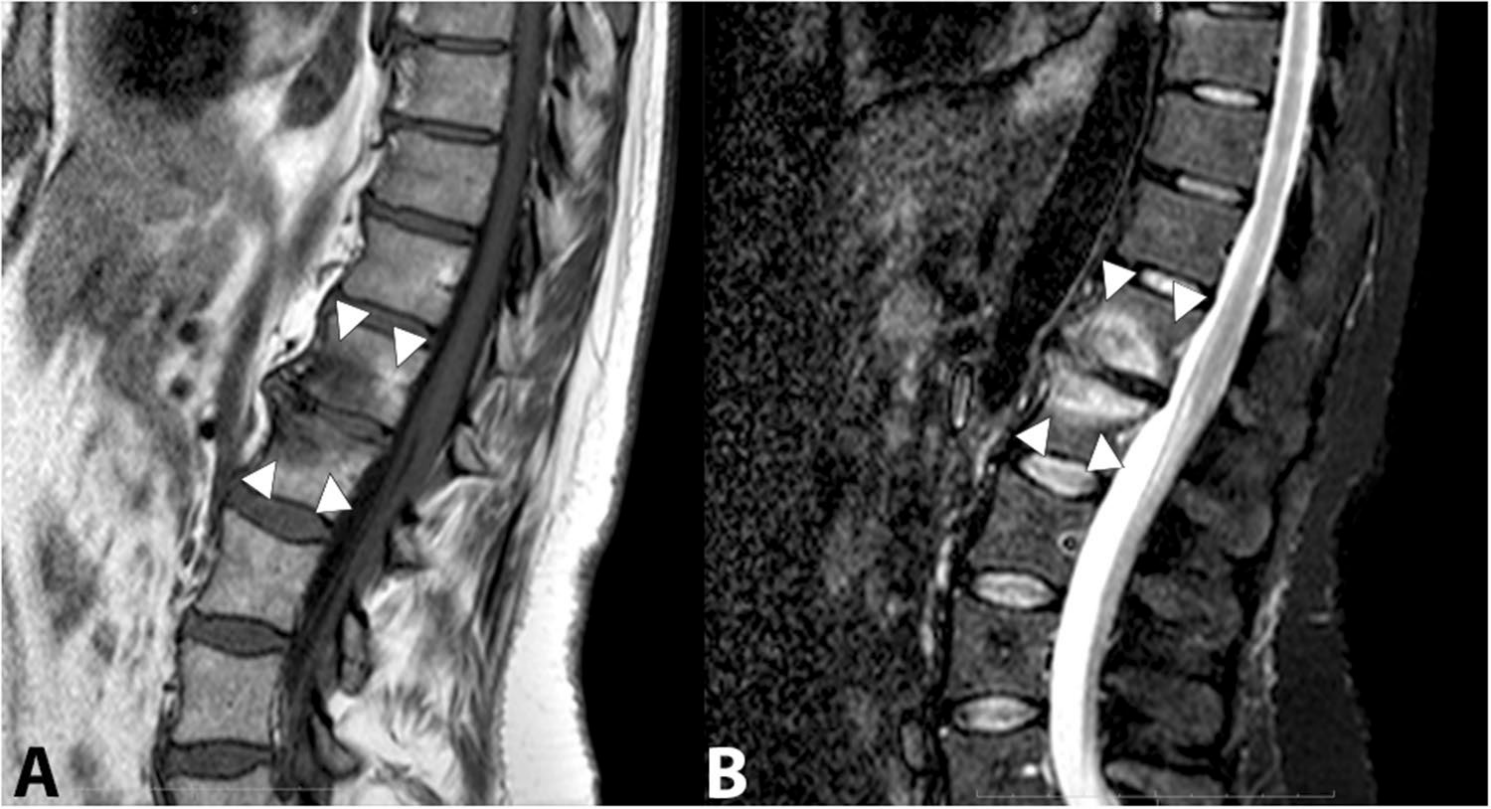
Sagittal T1-weighted image (a) and sagittal STIR image (b) showing fibrovascular and edematous pattern in the subchondral bone marrow of adjacent vertebral bodies L1/L2 (Modic type 1) (arrowheads). Bony degenerative changes can also be observed at the same level
